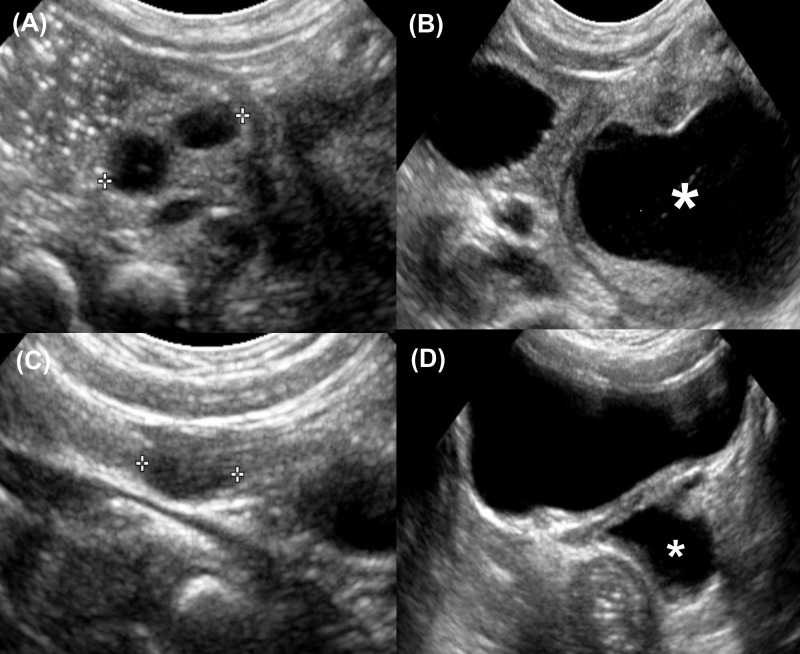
Natural course of OHVIRA syndrome with ectopic ureter insertion into obstructed hemivagina. (A),(B) Two days ultrasonography demonstrating 1.4cm sized ipsilateral ectopic multicystic dysplastic kidney(MCDK) and distended hemivagina. (C),(D) 14months ultrasonography demonstrating decreased size of ectopic MCDK (0.8cm) with decreased sized of distended hemivagina. *Asterisk: obstructed hemivagina
